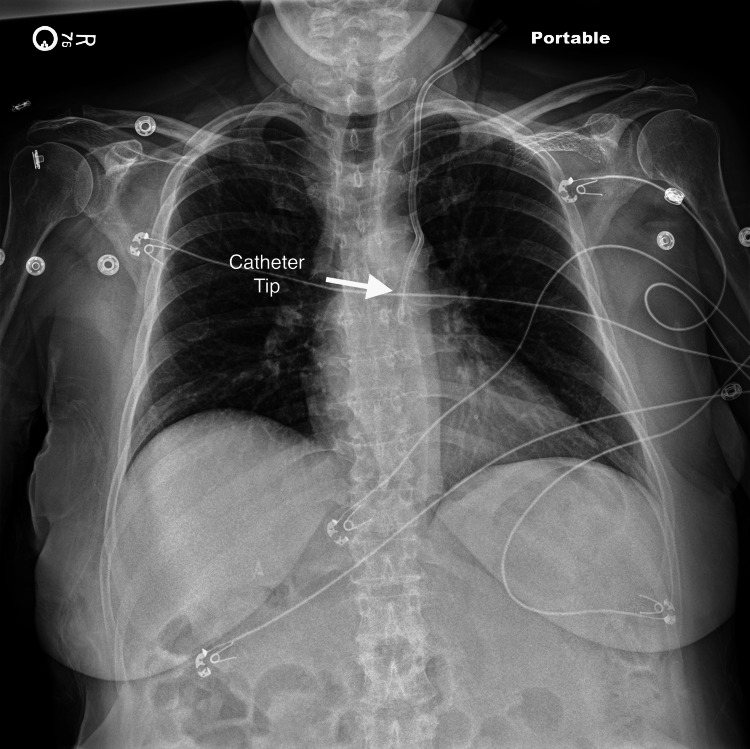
Chest X-ray post central line placement with catheter remaining on the left side of chest
